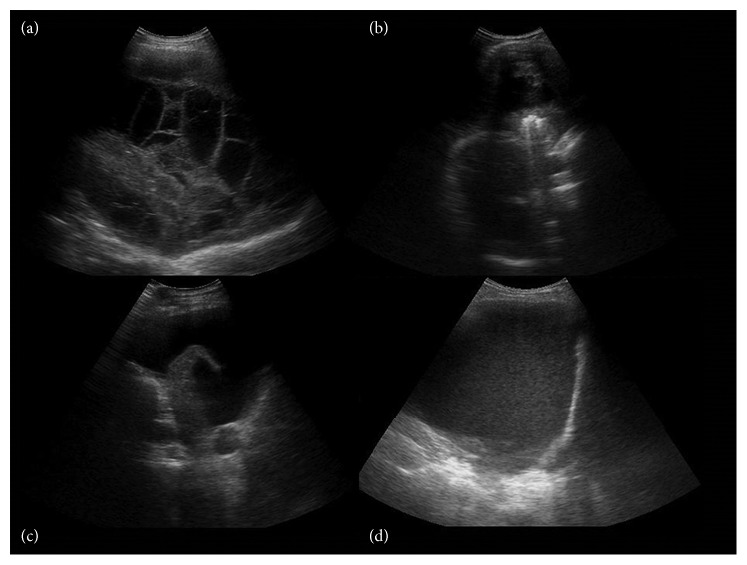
Representative sonographic findings for patients with different pleural effusions. Every chest US demonstrates the inner echo structure from complex septate (a) and nonseptate (b) to anechoic (c) and echogenic (d) pleural effusion.
